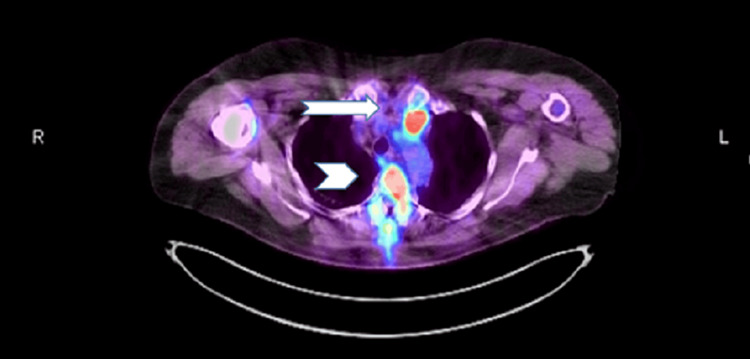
Left upper mediastinal mass (arrow) and vertebral metastasis (arrowhead) with high FDG uptake. FDG: fluorodeoxyglucose
